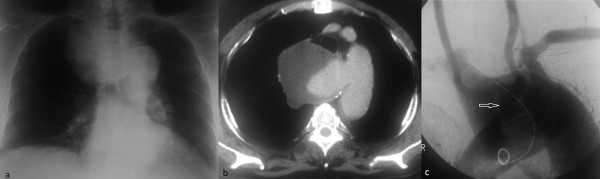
Pre-operative imaging demonstrating aneurysmal aberrant right subclavian artery. (a) Admission chest radiograph showing superior mediastinal mass (b) Computerised Tomographic images demonstrating CT a degenerative aneurysm of the ascending aorta and proximal left arch (c) Aneurysmal aberrant right subclavian artery.
